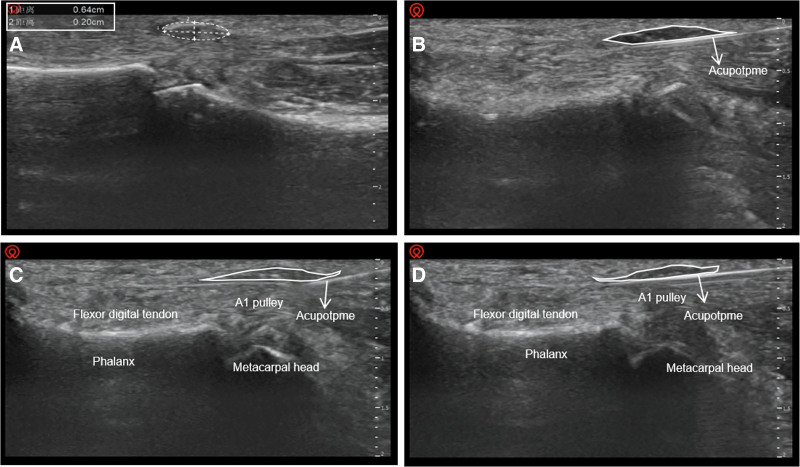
(A) The thickness of A1 pulley slip measured by ultrasound before treatment was 0.64 cm × 0.20 cm; (B) infiltration anesthesia with local injection under ultrasound; (C, D) ultrasound-guidance acupotome cutting path.
